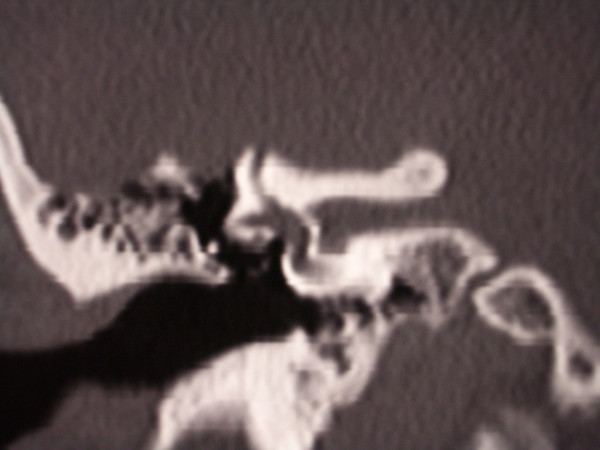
Pre-operative temporal bone coronal computed tomography images.
